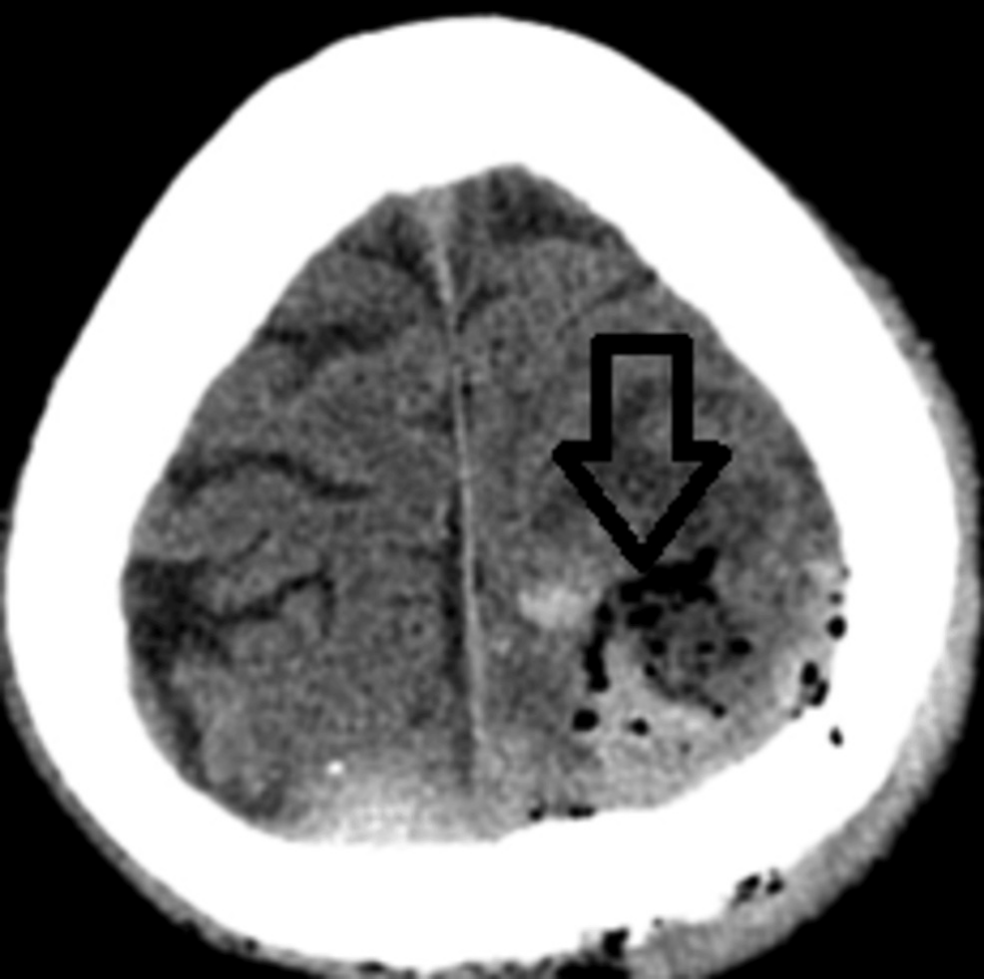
An MRI shows evidence of total extirpation of the tumor mass MRI: axial plane
